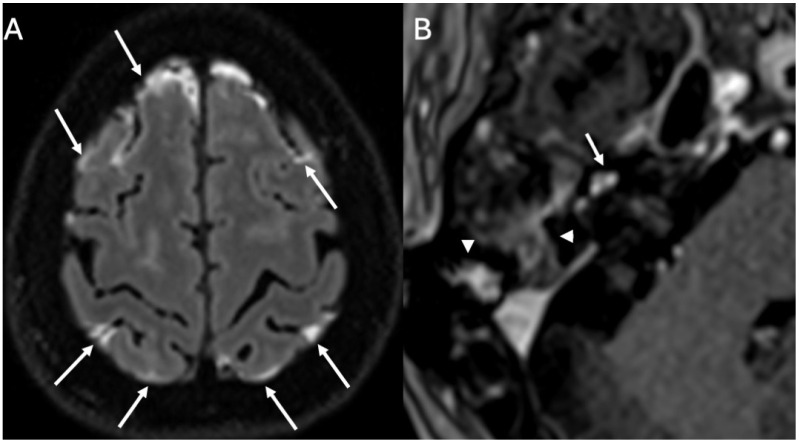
Acute Mastoiditis and labyrintitis in a 64-year-old patient with Hemophilus Influenzae Meningitis. Axial enhanced FLAIR (A) and T1 (B) show diffuse leptomeningeal enhancement (arrows in (A)), enhancement of the right mastoid (arrowheads in (B)) consistent with mastoiditis and enhancement of the right cochlea (arrow in (B)) due to labyrinthitis.
